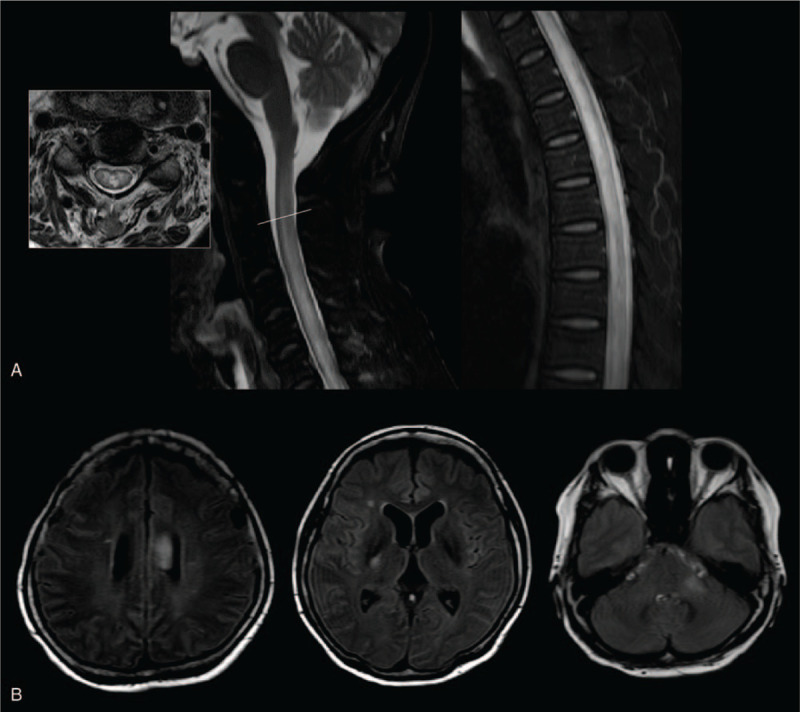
MRI of the spinal cord revealed T2 hyperintensity in whole spinal cord (A). And brain MRI showed the lesions in corpus callosum, right internal capsule and left cerebellar peduncle (B).
